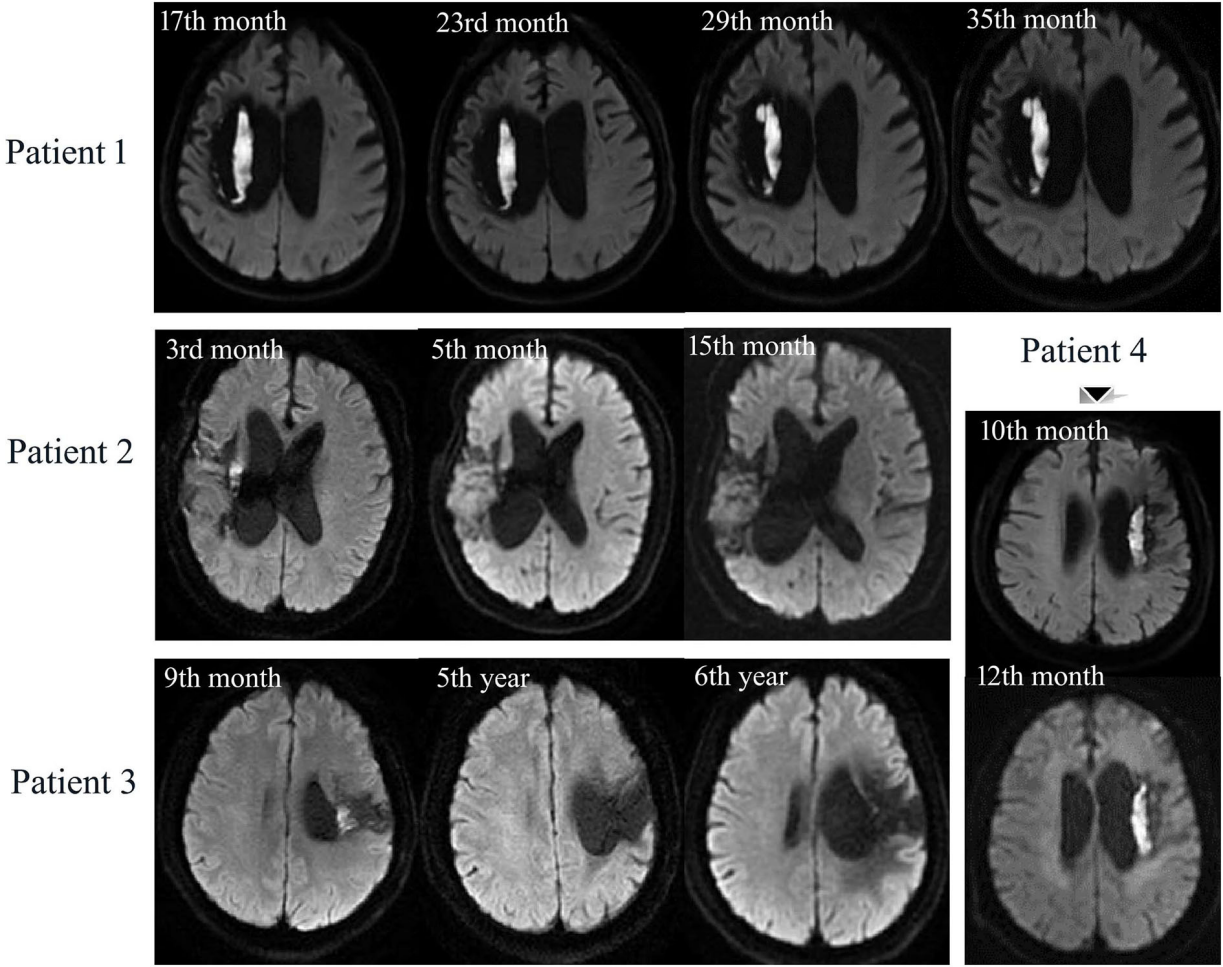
Longitudinal MRI scans of 4 DWI (+) patients with ICH–WS. Patient 1 has persistent DWI lesion during 18 months follow-up. Patient 2: DWI lesion is observable at the 3rd month, shrinks at the 5th month, and disappears at the 15th month. Patient 3: DWI lesion can be seen at the 9th month but disappears at the 5th and 6th year after ICH–WS. Patient 4 has an unchanged DWI lesion during 2 months follow-up.
